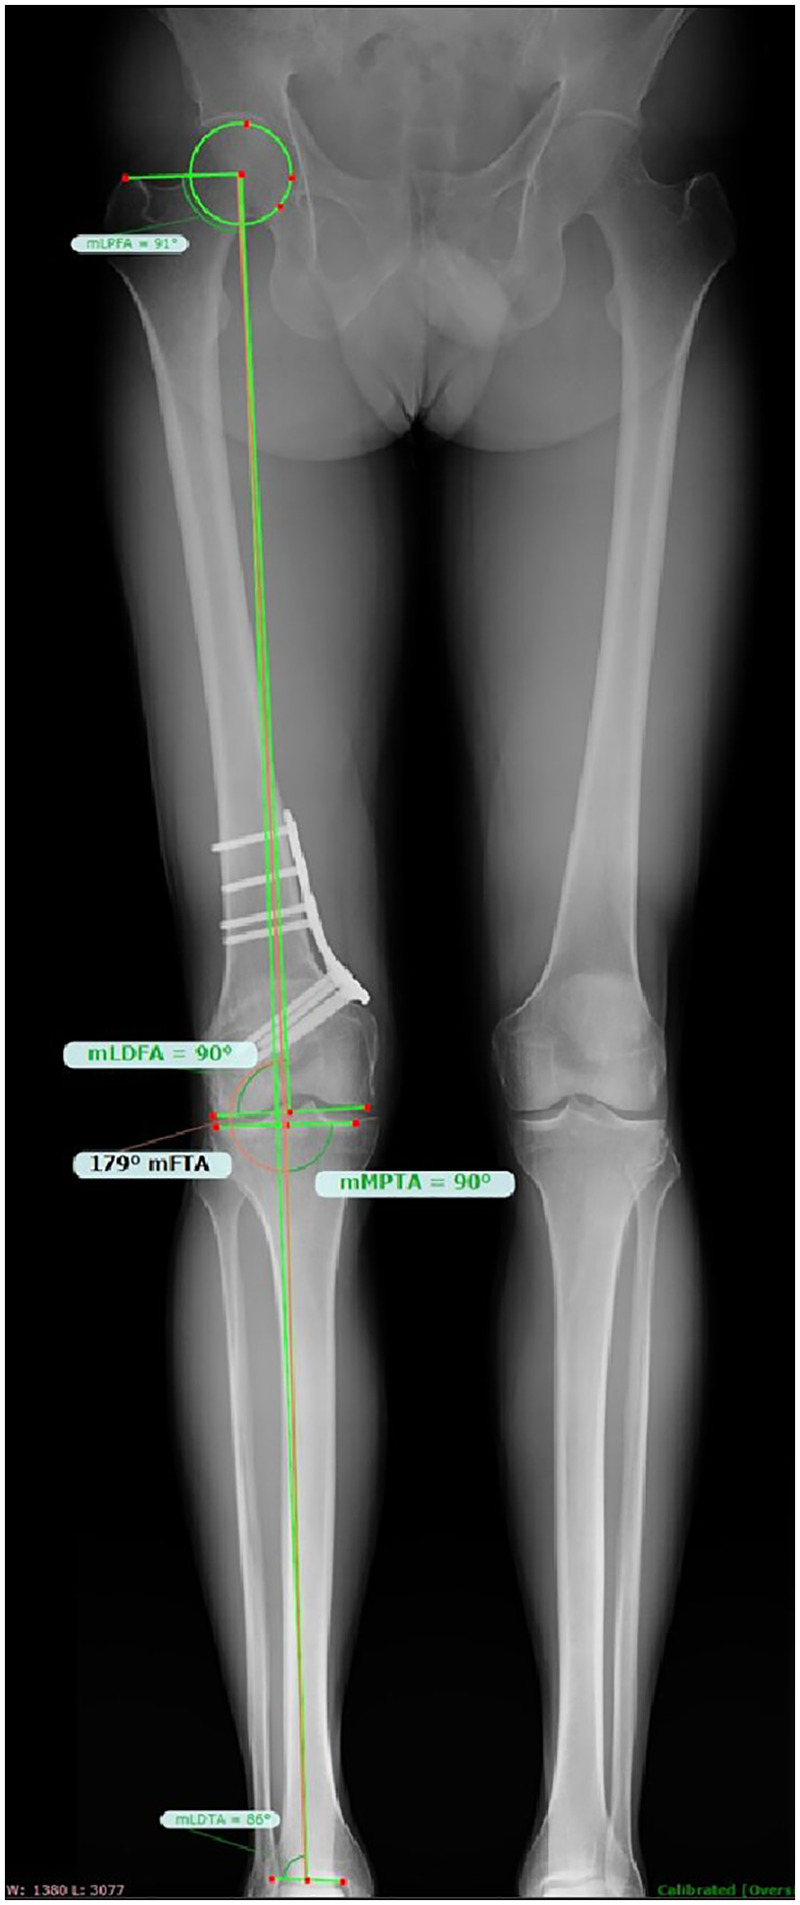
Postoperative Re-evaluation on full-length x-ray.
